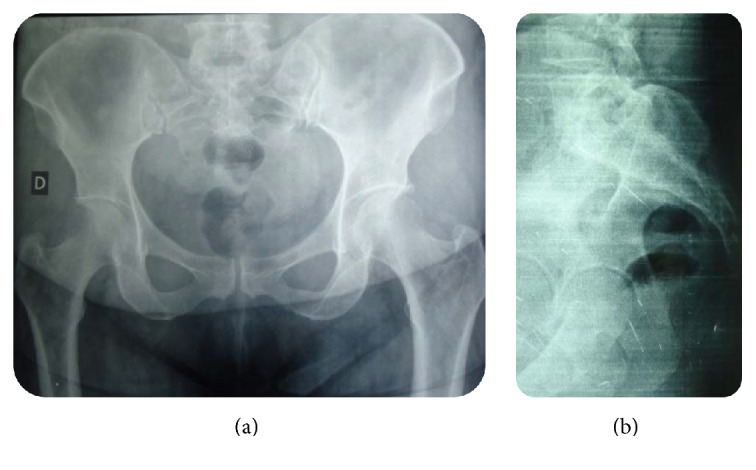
Follow-up AP and oblique X-rays of pelvis at 3 years showing no evidence of recurrence.
